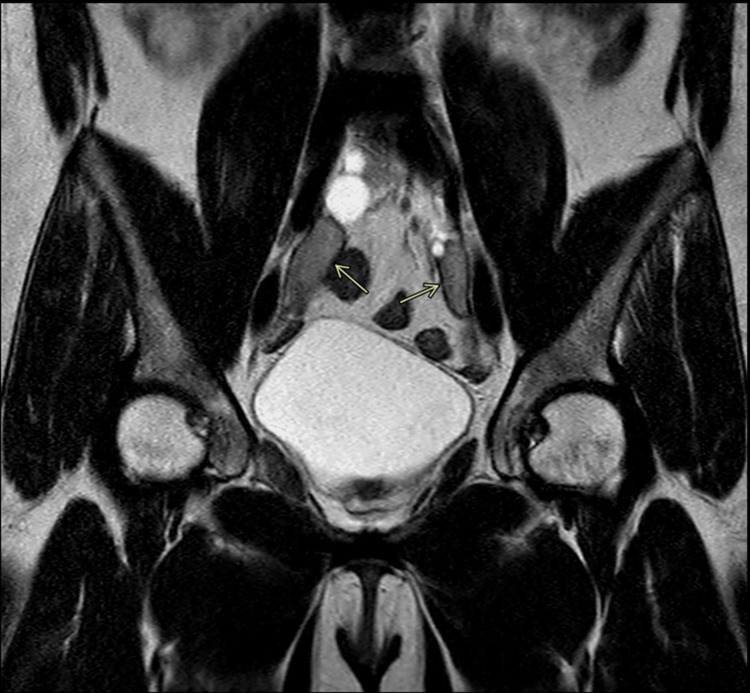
Abdominal MRI revealed oval-shaped, well-defined solid images adjacent to the bilateral iliac vessels, measuring 2.2 x 1.7 cm on the right and 2.4 x 1.0 cm on the left, potentially corresponding to male gonads
